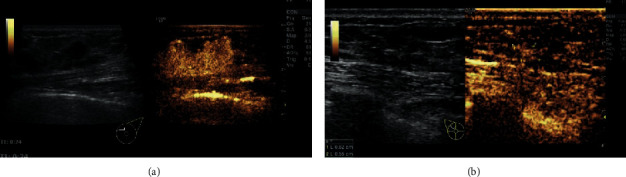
Contrast-enhanced ultrasound measurement of nonluminal breast cancer with nonconcentric shrink mode before and after NAC. (a) Left, two-dimensional ultrasound image of the lesion before NAC; right, ultrasound contrast image of the lesion before NAC; (b) Left, two-dimensional ultrasound image of the lesion after NAC; right, ultrasound contrast image of the lesion after NAC.
